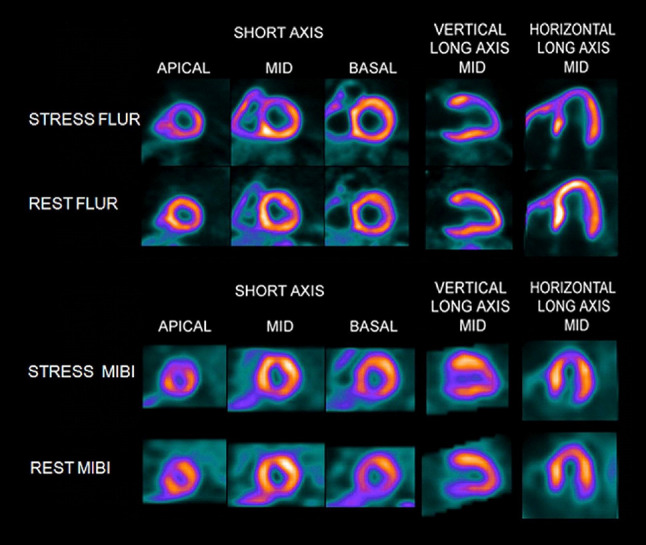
FLUR PET and MIBI SPECT images from an 82-year-old man. The FLUR PET (top) and MIBI SPECT (bottom) images from an 82-year-old man with shortness of breath and an occluded native proximal left anterior descending (LAD) coronary artery and an occluded left internal mammary graft to the LAD and no other significant native CAD. The FLUR images show a severe reversible perfusion defect throughout the territory of the occluded proximal LAD, whereas the MIBI images show only a moderate perfusion defect in the distal LAD territory (apical slices). FLUR = Flurpiridaz F 18; MIBI = Tc-99m sestamibi. Reprinted from the Journal of the American College of Cardiology (JACC), 61(4), Daniel S. Berman, Jamshid Maddahi, B. K. Tamarappoo, Johannes Czernin, Raymond Taillefer, James E. Udelson, C. Michael Gibson, Marybeth Devine, Joel Lazewatsky, Gajanan Bhat, Dana Washburn, Phase II safety and clinical comparison with single-photon emission computed tomography myocardial perfusion imaging for detection of coronary artery disease: flurpiridaz F 18 positron emission tomography, 469-77, Copyright (2013), with permission from Elsevier [41]
